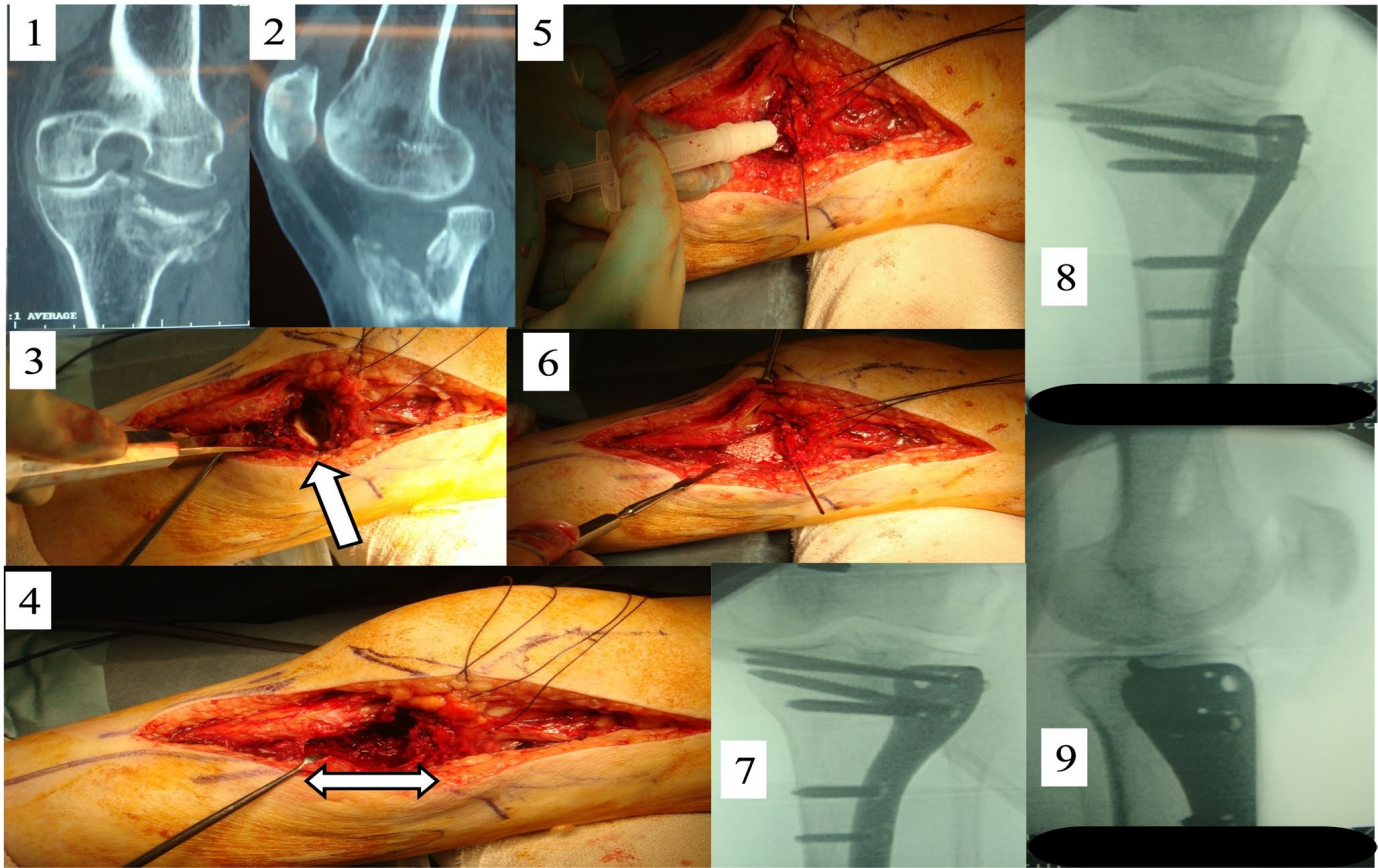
Surgical administration of KUR-111: 1. Axial CT image of a type II tibial plateau fractue in an elderly patient. 2. Sagittal CT image of a type II tibial plateau fractue in an elderly patient. 3. Intra-operative image following a lateral approach with arrow showing degree of depression of articular surface prior to reduction. 4. Intra-operative image with arrow showing degree of bone void created after reduction of articular surface. 5. Intra-opertaive image showing delivery of KUR-111 graft material to the bone void. 6. Intra-operative image showing filling of the void with the KUR-111 graft material. 7,8. AP intraoperative radiographic images showing the result of reconstruction. 9. Lateral intraoperative radiographic image showing the result of reconstruction
